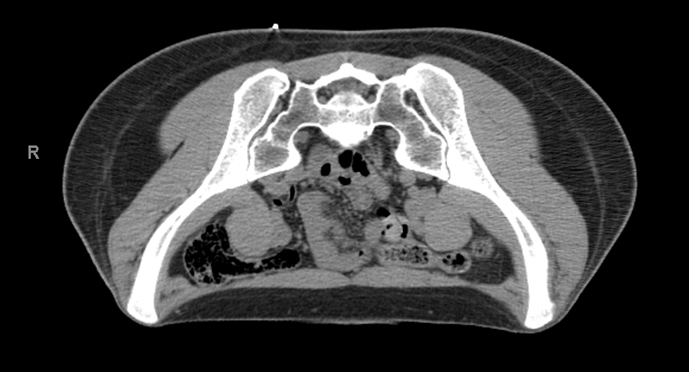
Sacral lesion is occult on CT scan. A representative axial CT image (120 kVp, 90 mA, slice thickness 3 mm) of the expected location of the intraosseous hibernoma does not demonstrate an appreciable abnormality.
